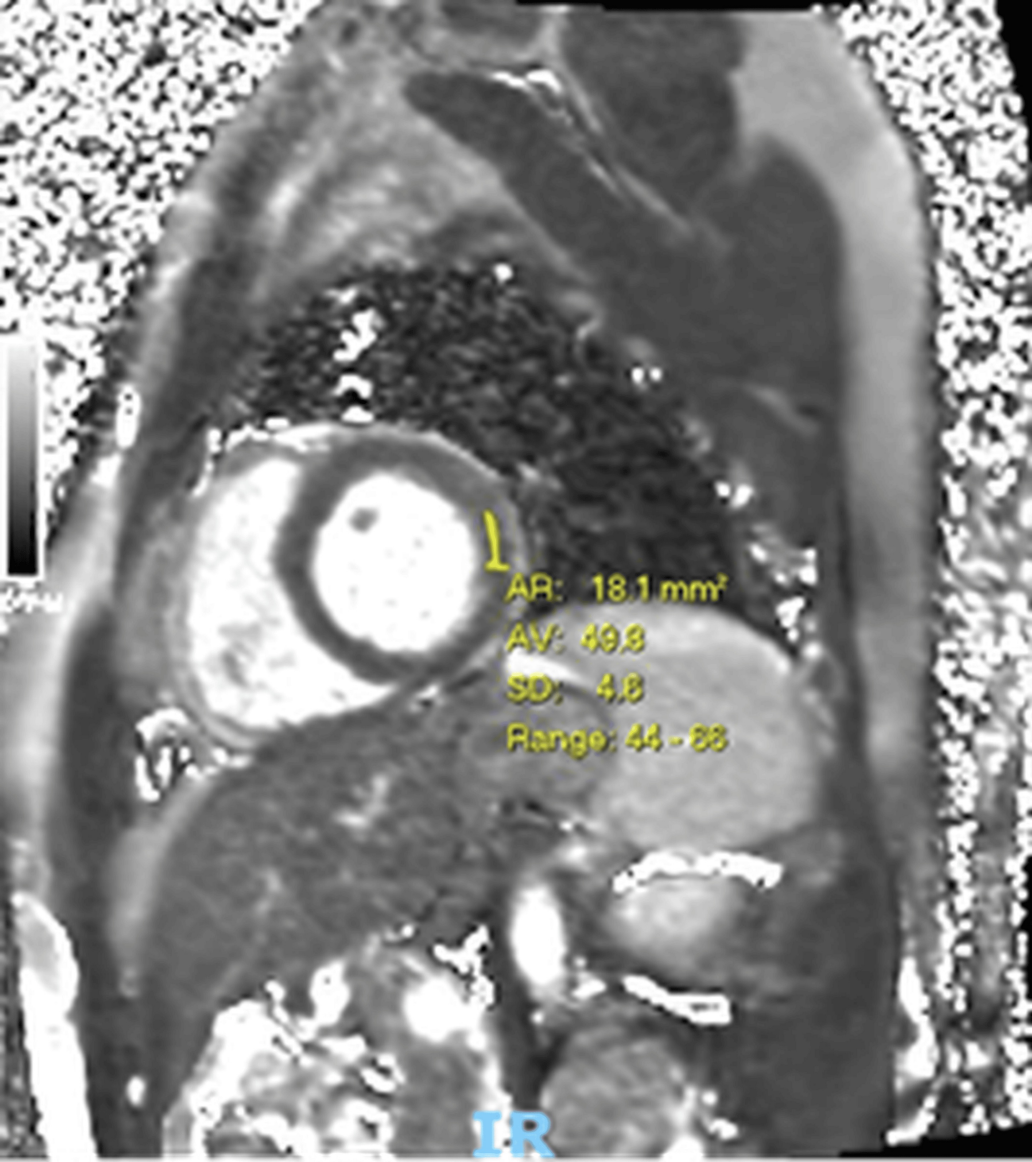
Follow-up cardiac MRI demonstrating resolution of myocardial oedema Three months after discharge, cardiac MRI T2 mapping demonstrates normalized or near-normalized myocardial T2 values (average: 49.9 ms; reference range: 44–68 ms). The restoration of T2 values to the normal range indicates resolution of myocardial oedema, consistent with recovery from the acute inflammatory phase of myocarditis.
